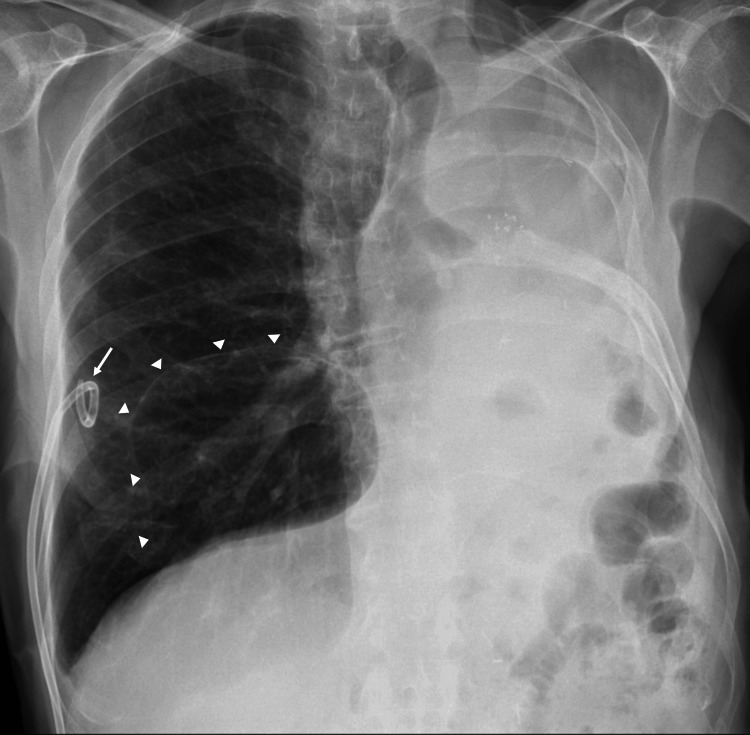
Chest radiograph demonstrating complete re-expansion of the right lung. Chest radiograph demonstrating full re-expansion of the right lung following resolution of the air leak. Arrowheads indicate the middle-lobe bulla seen on the CT scan, and the arrow denotes the pigtail pleural drainage catheter.
